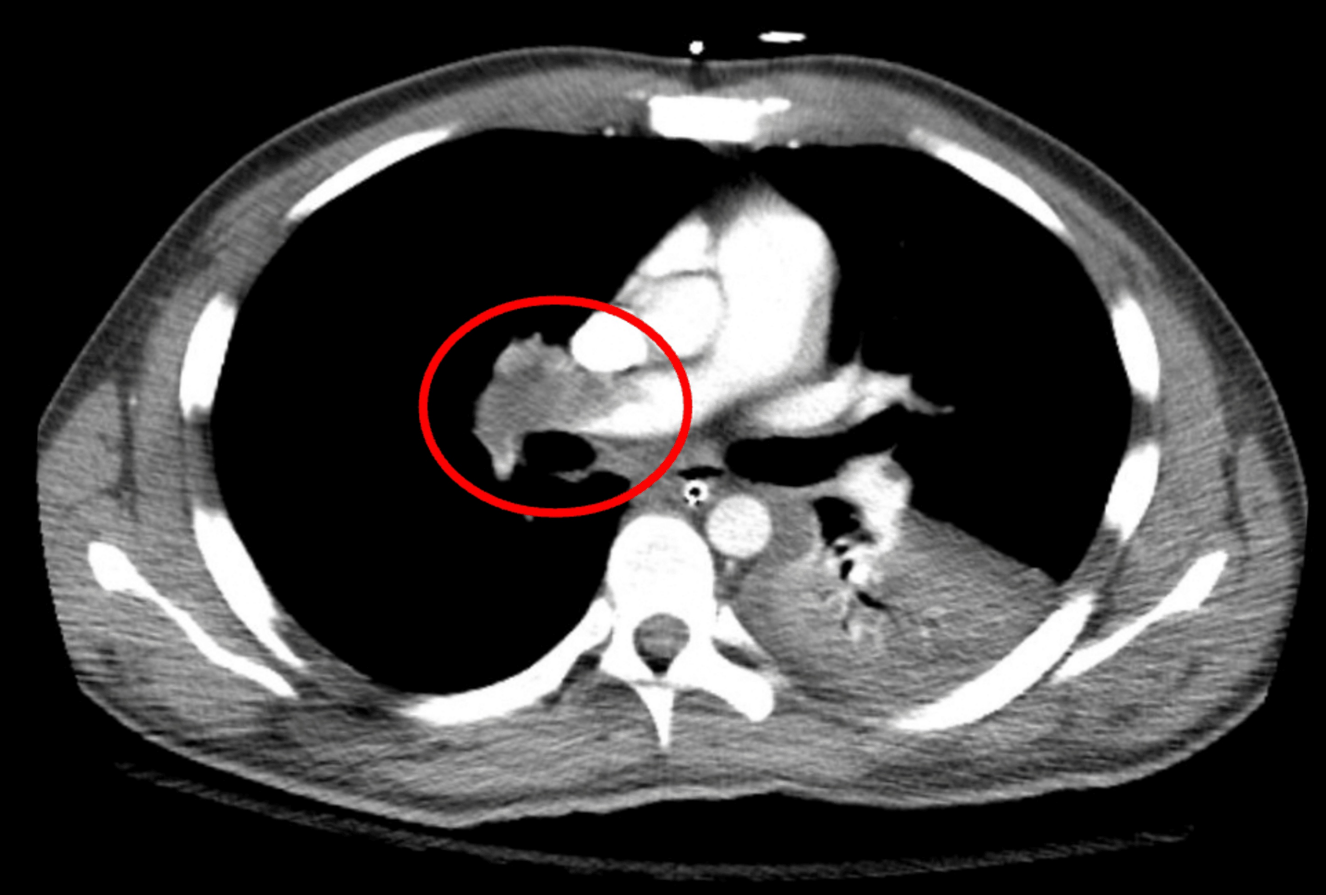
CT angiogram CT angiography of the chest showing large pulmonary emboli in the right pulmonary artery branch. The embolism is circled in red.
